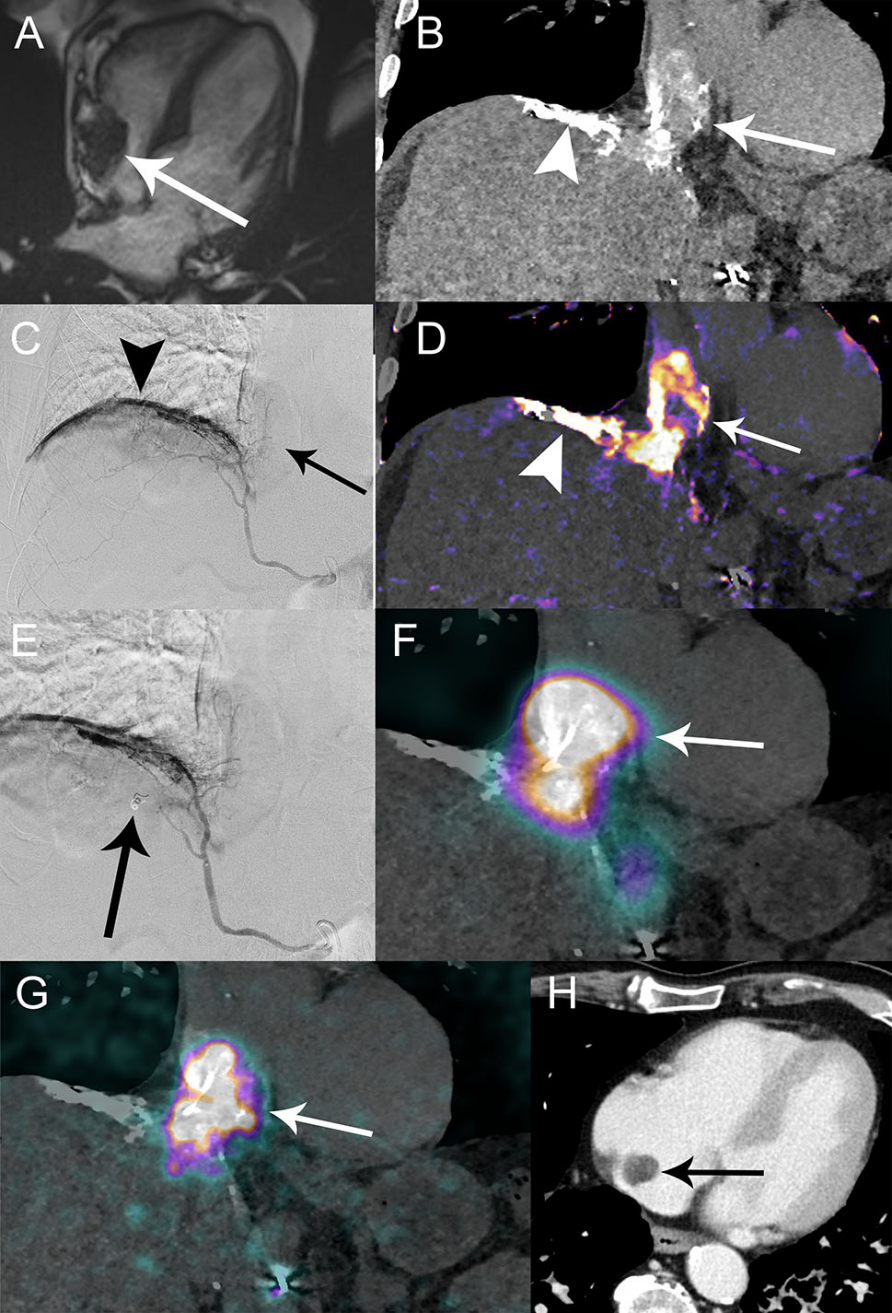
A Pre-treatment cardiac MRI: right atrium tumor extension (5.1 × 3 cm) (arrow). B–D Pre-treatment angio-CT (B), digital subtraction angiography (DSA) (C) and subtraction CT (D) obtained with the injection of iodinated contrast through a microcatheter (Progreat 2.7 Fr, Terumo) positioned in the right inferior phrenic artery (rIPA) shows contrast uptake of the atrial tumor (arrows) and diaphragm (arrowheads). E DSA: microcoil (Tornado 0.018 inch/3–2 mm, Cook) embolization (arrow) of the posterior branch of rIPA to protect as much diaphragm as possible and prioritize the blood flow to the tumor. Pre-treatment angio-CT fused with F
